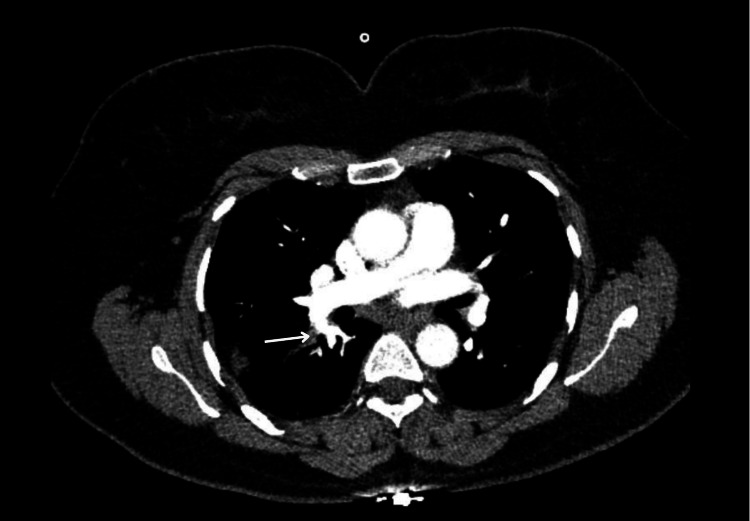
Axial CT pulmonary angiography (CTPA) image from Case 2 demonstrating a filling defect in the segmental pulmonary artery branch supplying the superior part of the right lower lung lobe (arrow)
